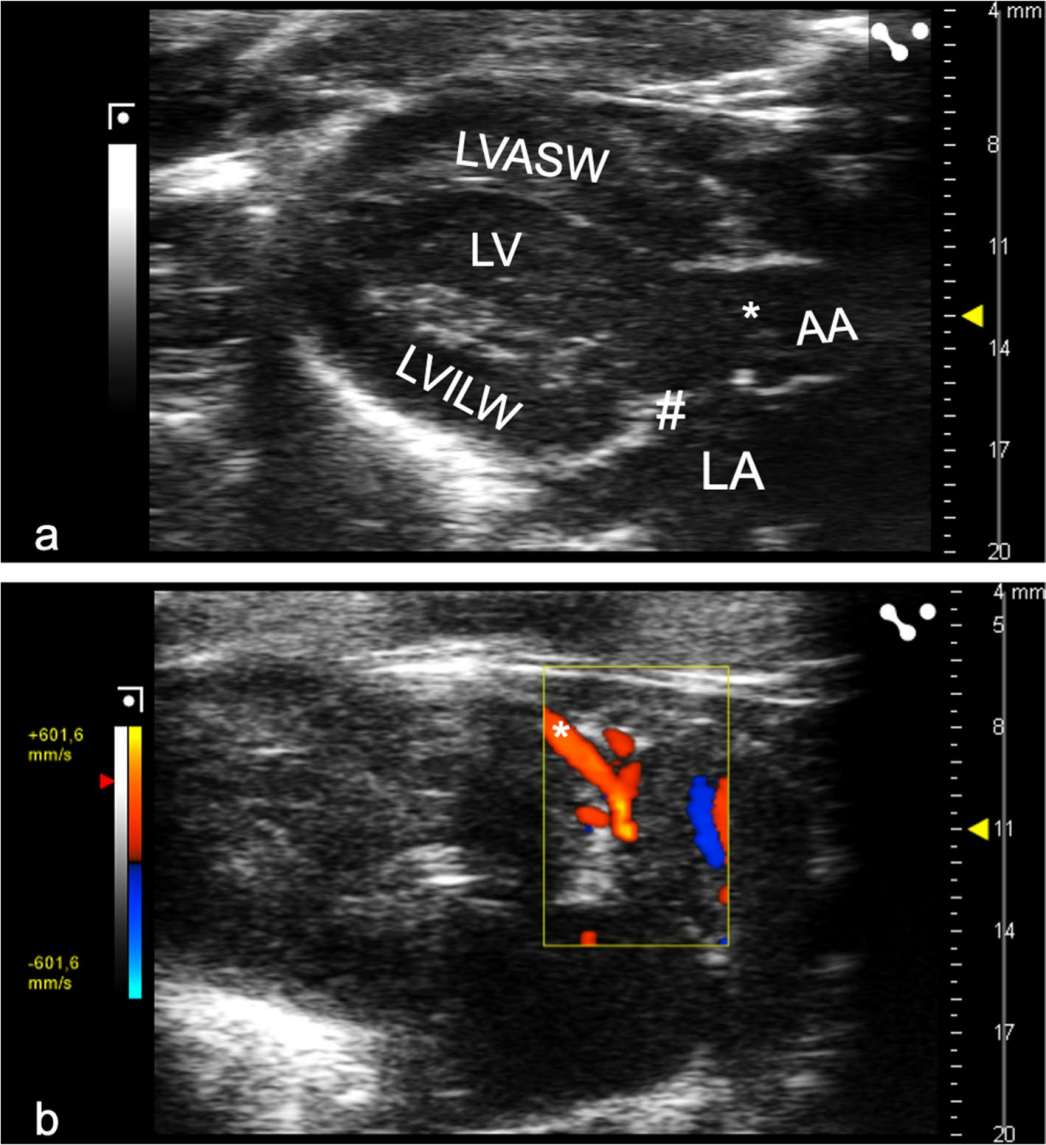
Representative B-mode and colour Doppler images acquired in a parasternal long axis (PLAX). a B-mode PLAX of the left ventricle. These images were used for volumetric and perfusion measurements. *AA* Ascending aorta, *LA* Left atrium, *LV* Left ventricle, *LVASW* Left ventricular anteroseptal wall, *LVILW* Left ventricular inferolateral wall, *aortic valve, *#* mitral valve. b Exemplary colour Doppler image of the proximal left coronary artery, which was assessed by pulsed-wave Doppler in the following step. *Proximal left coronary artery
